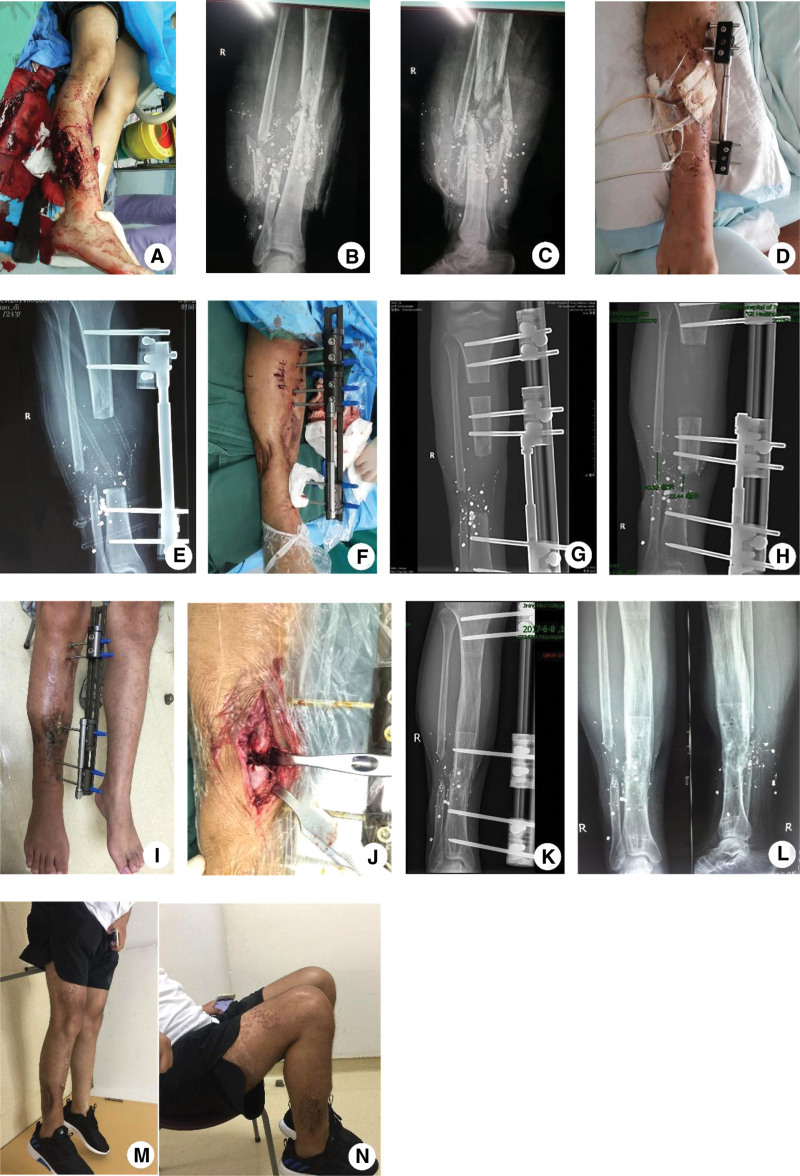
(A, B, C) A 24-yr-old man sustained open injuries in the right lower limb caused by gunshot. (D, E) Debridement, temporary fixation of external fixator, and VSD of the wounds were carried out. (F, G, H) A Ilizarov unilateral external fixator was used and bone transport were performed, and the length of the tibial defects was approximately 10 cm. (I, J) The docking sites did not heal after bone transport. The docking sites were cleared and cancellous bone grafted. (K) At 4 mo after bone graft, X-ray examination showed healing of the bone fracture, and the external fixator was scheduled to be removed. The postoperative external fixation time was 20 mo, and the external fixation index was 2.0. (L, M, N) Clinical appearance and X-ray findings 4 mo after external fixator was removed. The postoperative ASAMI functional score of the affected limb was excellent. ASAMI = Association for the Study of the Method of Ilizarov, VSD = vacuum sealing drainage.
